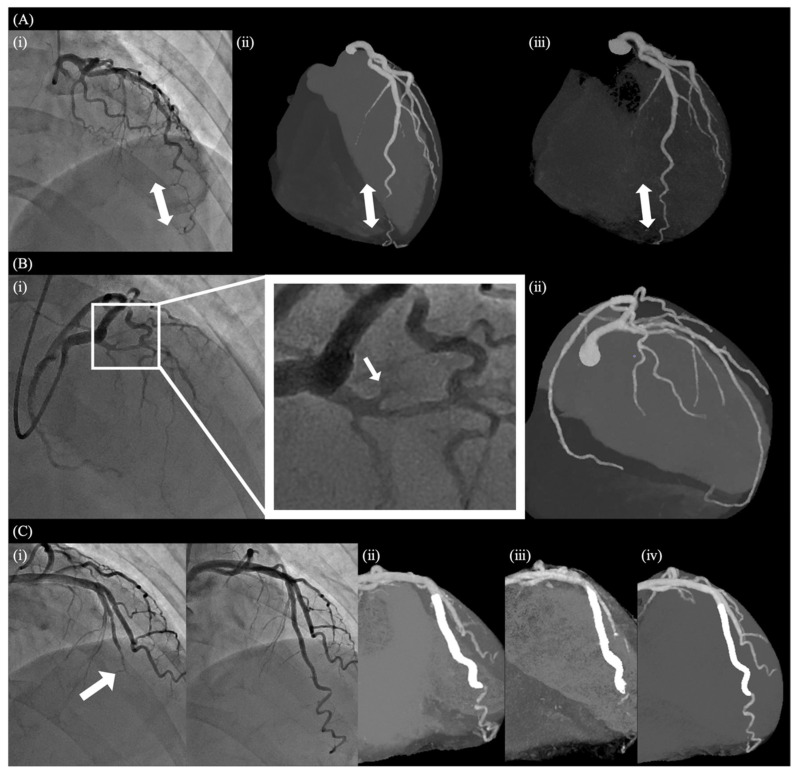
Follow-up CCTA after ICA or treatment in SCAD. (A) Type 3 SCAD in the distal LAD. (i) Arrow indicates the site of the lesion on admission. (ii) At 1-month follow-up CCTA after ICA, the dissection persists (arrow). (iii) Healing is observed at 7-month follow-up (arrow). (B) Type 4 SCAD in the proximal LAD. (i) Arrow denotes the occlusion site at presentation. (ii) CCTA performed 1 week after ICA demonstrates restoration of flow in the previously occluded vessel. (C) A case of type 4 SCAD in the mid-LAD. (i) Arrow marks the lesion site at presentation. The lesion was treated with PCI and DES implantation. Residual dissection is observed distal to the stent. (ii–iv) Serial CCTA images for follow-up of the lesion. At 1 week after PCI (ii) and at 3 months after PCI (iii), residual dissection persists distal to the stent. (iv) At 8 months after treatment, progressive healing is observed, with resolution of the dissection. Abbreviations: ICA = invasive coronary angiography; CCTA = coronary computed tomography angiography; PCI = percutaneous coronary intervention; DES = drug-eluting stent.
